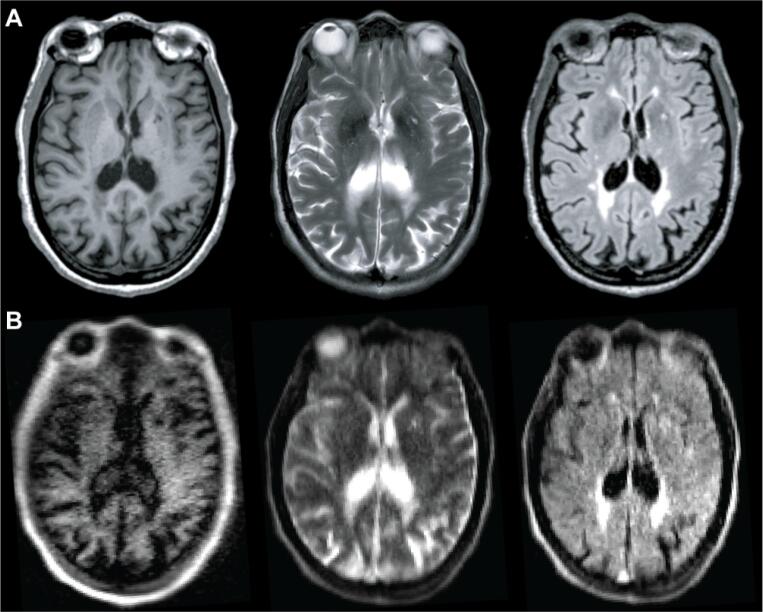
MS lesions on 3T and 64mT pulse sequences. Paired 3T (A) and 64mT (B) images from a 66-year-old woman with stable RRMS. Sequences include T1w (left), T2w (center), and T2-FLAIR (right). Images from both scanners show deep gray matter lesions and periventricular white matter lesions. Note also the superior sagittal sinus is hyperintense in the 64mT T2w and T2-FLAIR sequences, but not at 3T.
